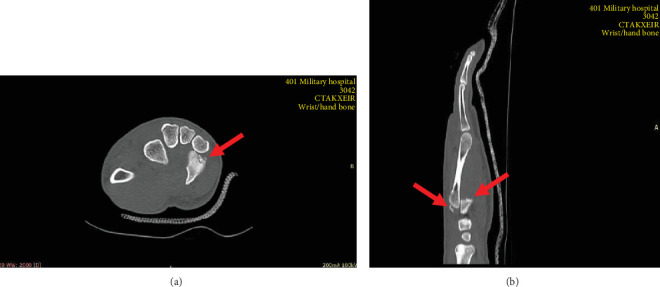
(a, b) CT images showing the dorsal dislocation of the fifth CMC joint and a bony fragment at the base of the fifth metacarpal that originated from the hamate (red arrows).
